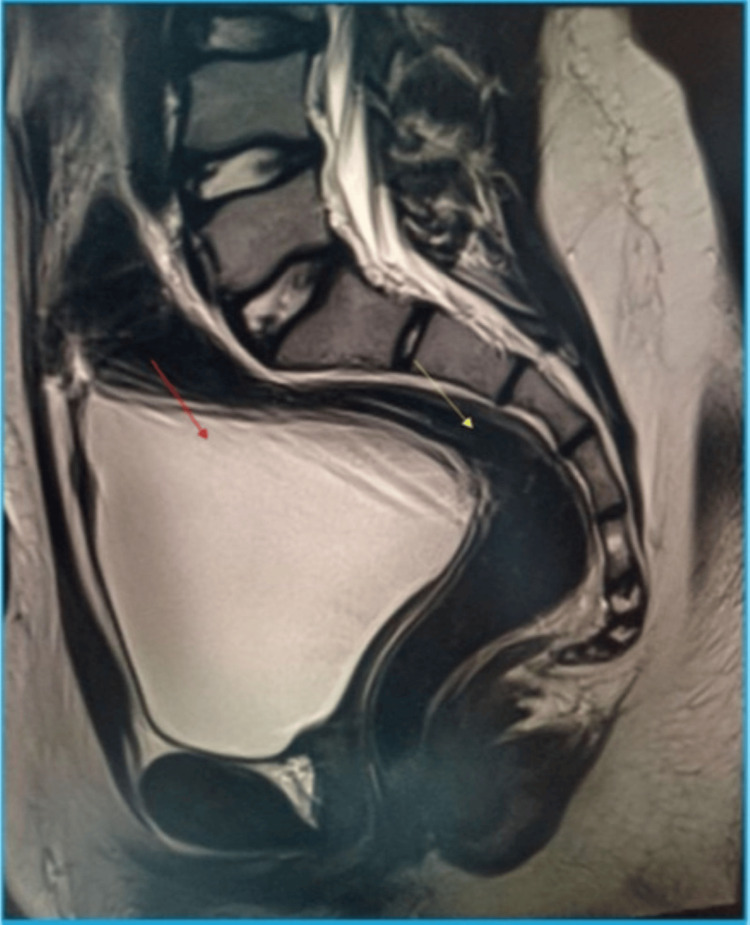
Abdominopelvic MRI. Sagittal MRI section demonstrating absence of the uterus. The urinary bladder (red arrow) is seen anteriorly, and the rectum (yellow arrow) posteriorly. MRI, magnetic resonance imaging.
